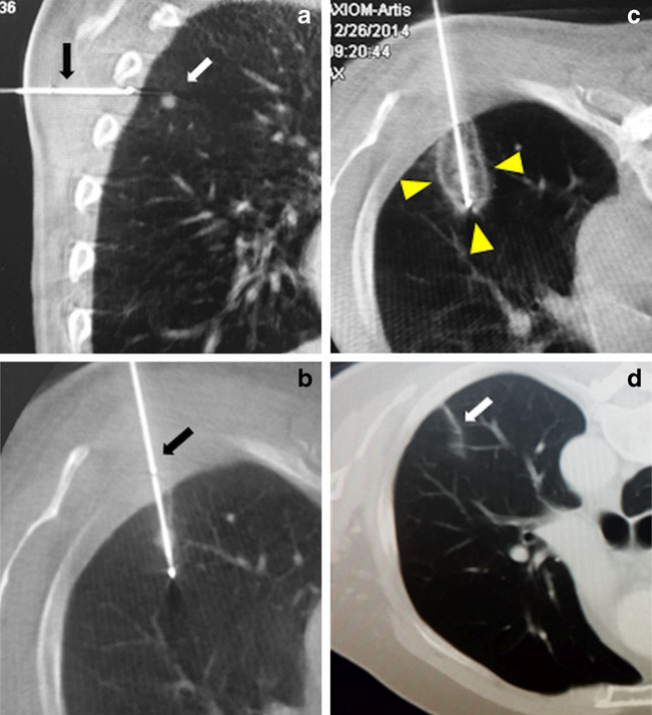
Cryoablation of a lung metastasis. a The sagittal cone beam CT image shows a 7-mm biopsy-proven colon cancer metastasis (*white arrow*) and the cryoablation needle (*black arrow*). b Under local anaesthesia, the cryoablation needle is percutaneously inserted into the lesion (*black arrow*). c During ablation, an iceball (*arrowheads*) is formed that covers the lesion. d After 1 year, the control CT image shows a fibrotic remnant (*white arrow*) in place of the tumour
